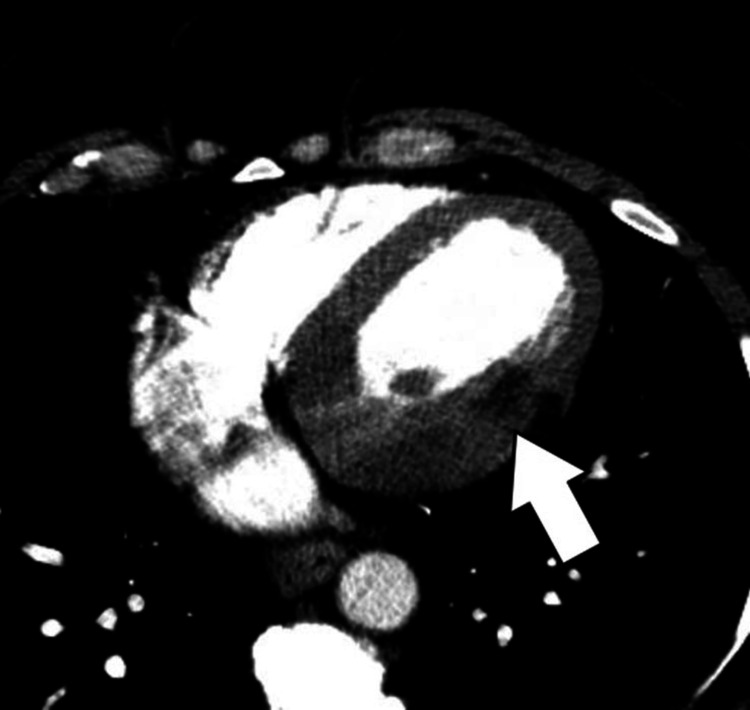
Contrast CT angiogram of the chest A selected axial view shows regions of fat attenuation (low HU) in the epicardial mid-lateral wall and base of the anterolateral papillary muscle, the same location as the cardiac magnetic resonance images (arrow).
